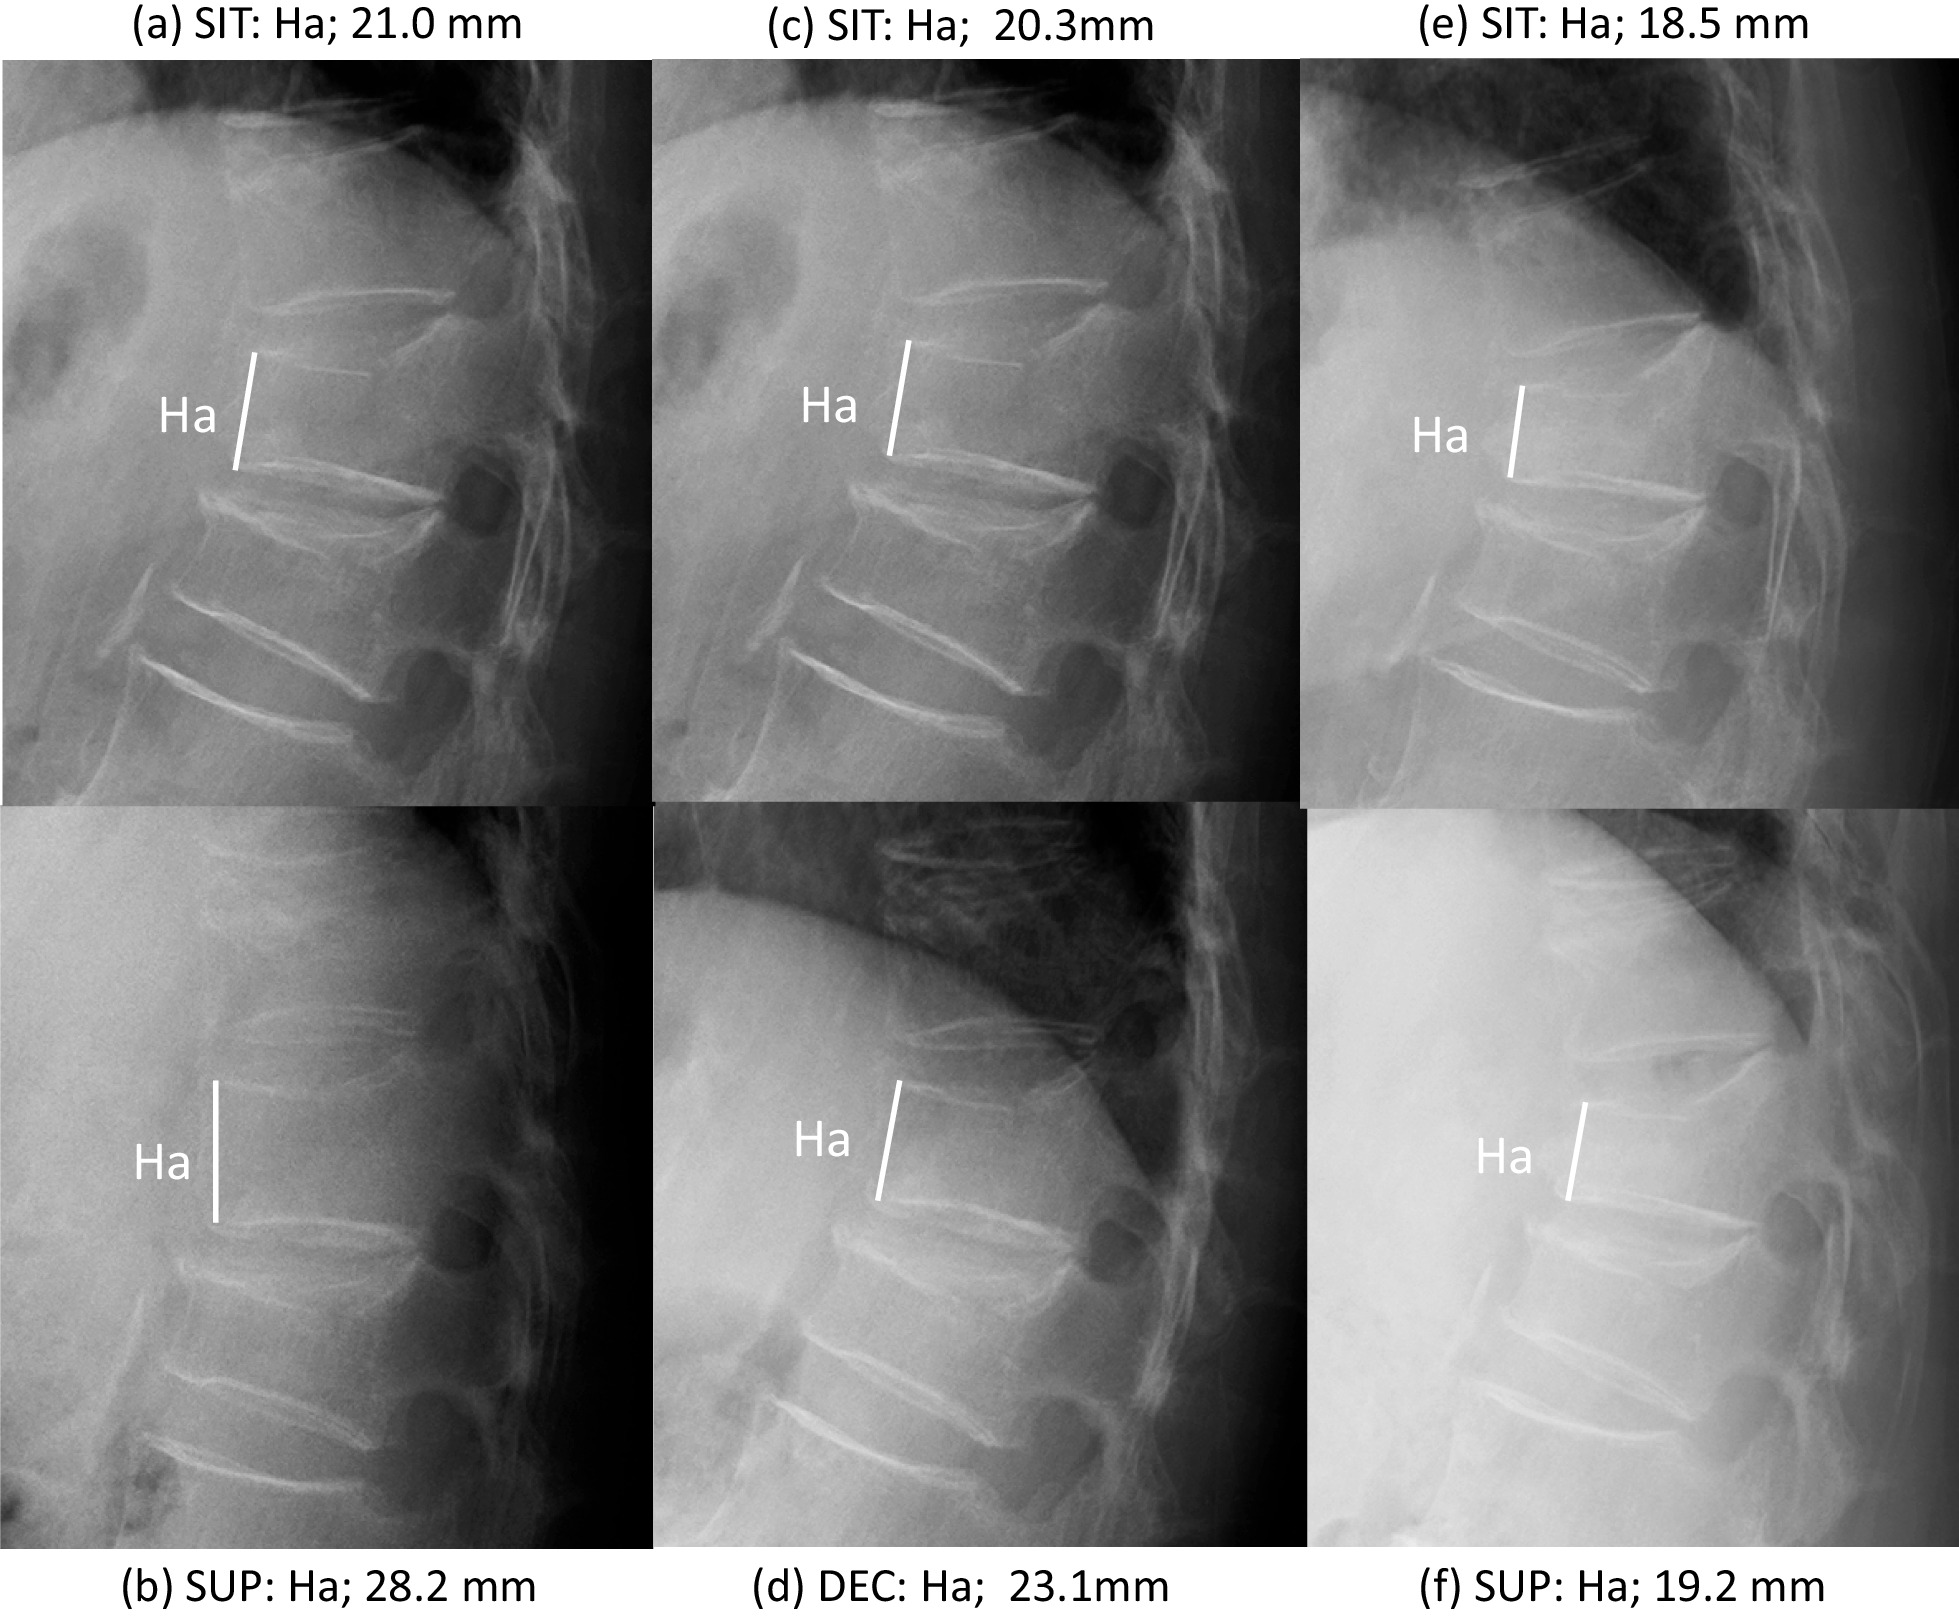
Radiological evaluation of one patient with a fresh OVF at T12 and a stable previous OVF at L1. (a, b) Lateral radiographs obtained in SIT and SUP to evaluate V-mobility in the initial evaluation at 13 days after OVF onset. (c, d) Radiographs obtained during treatment in SIT and DEC to show V-mobility at 61 days after OVF onset. (e, f) Radiographs obtained in SIT and SUP in the final evaluation to detect radiological bone union at 146 days after OVF onset. *OVF* osteoporotic vertebral fracture, *SIT* sitting position, *SUP* supine position, *DEC* lateral decubitus position, *V-mobility* vertebral mobility
